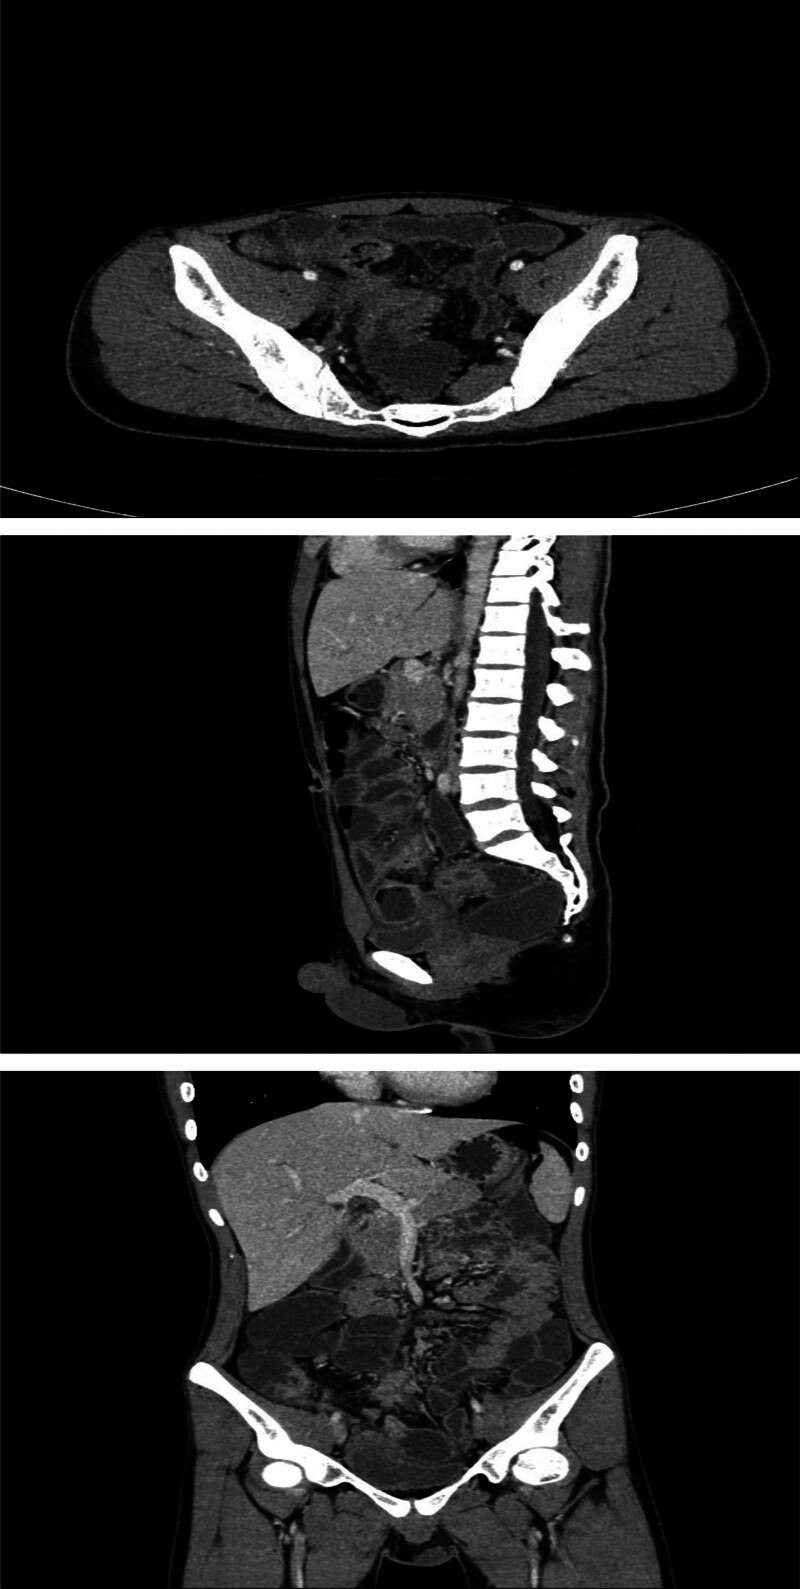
CT manifestations of the small bowel on review after treatment of Crohn’s disease combined with bladder fistula. CT = computed tomography.
